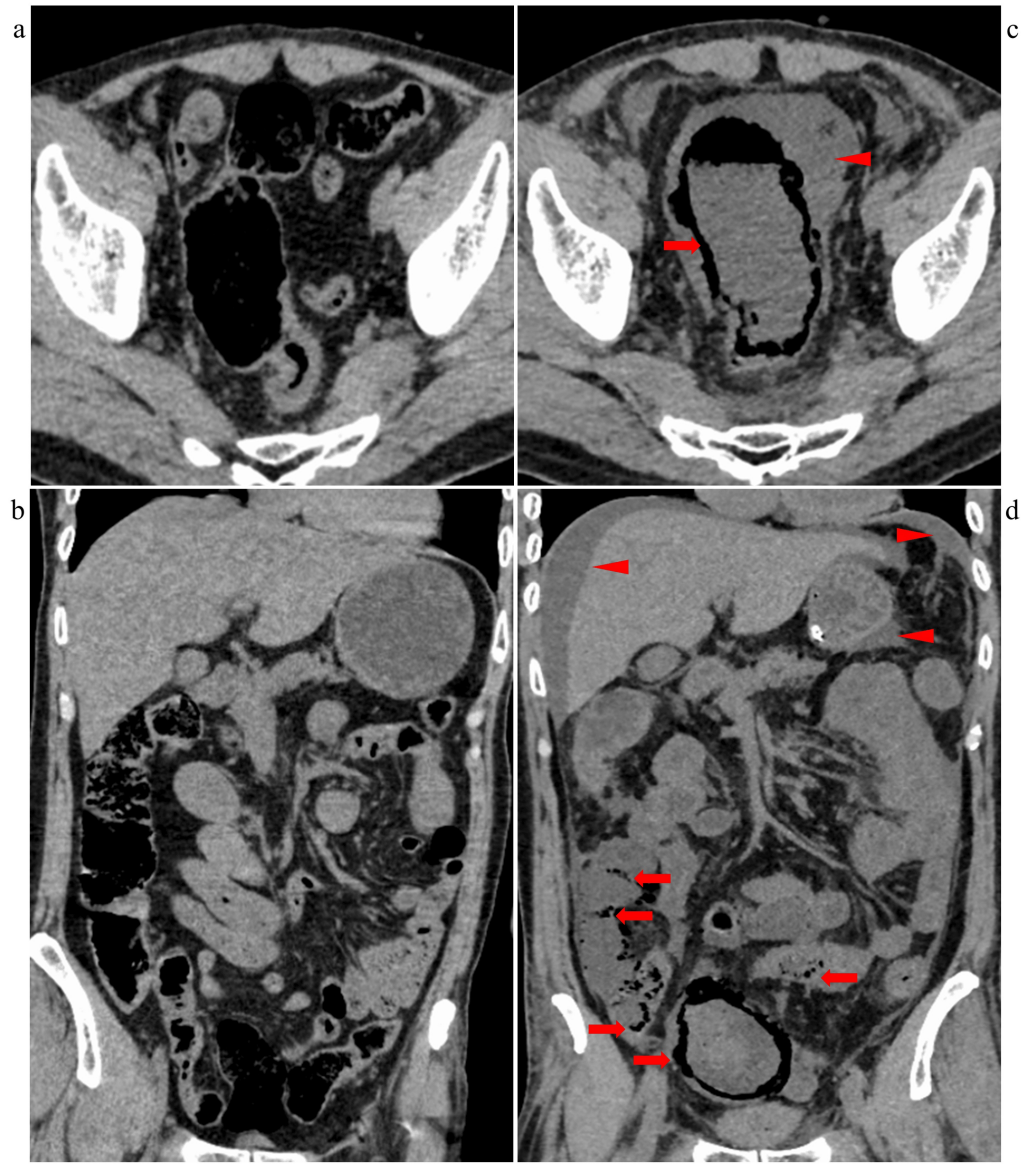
Computed Tomography Imaging of the Abdomen and Pelvis Computed tomography imaging of the abdomen and pelvis obtained on admission (a, b) and 36 hours after admission (c, d). Panels (a) and (c) show axial views, while panels (b) and (d) show coronal views. Extensive pneumatosis intestinalis (arrows) was observed from the rectum to the sigmoid colon, with additional involvement of the terminal ileum, suggesting non-occlusive mesenteric ischemia (c, d). Massive ascites (arrowheads) was also noted (c, d).
